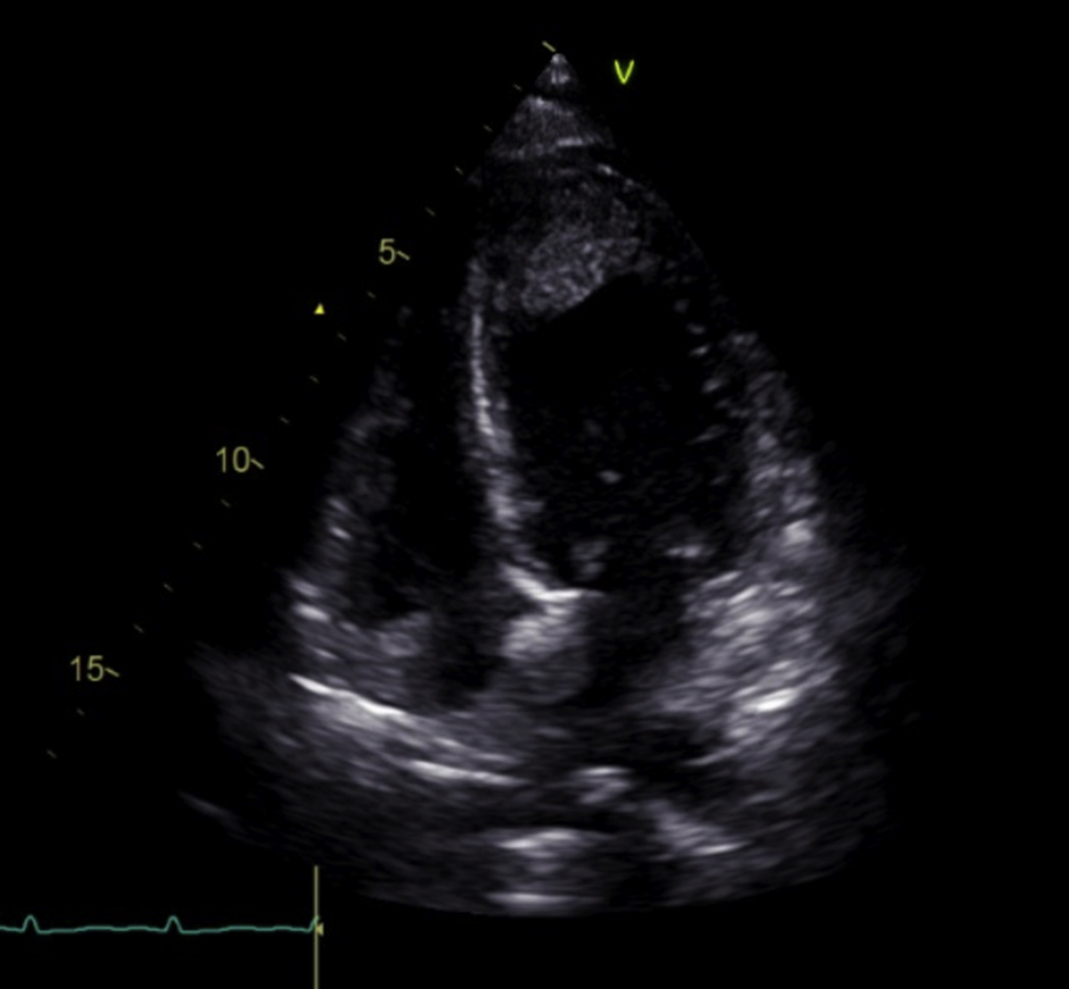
Still Image From Echocardiography on Admission Apical 4-chamber view showing global hypokinesia and apical thrombus.
